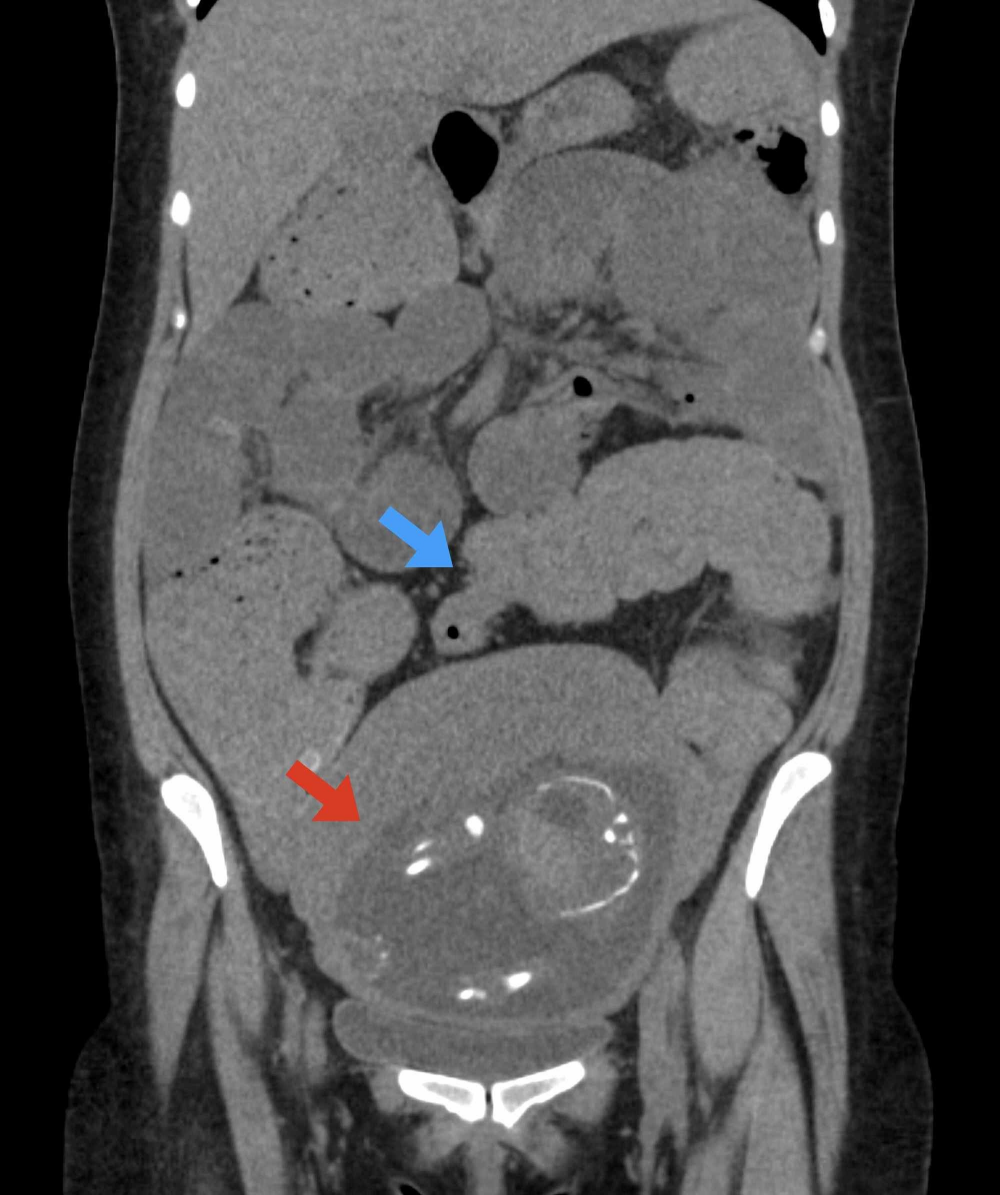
Coronal reconstruction from CT scan of the abdomen and pelvis demonstrating colonic distention with abrupt transition to complete collapse in the region of the sigmoid colon (blue arrow) and gravid uterus (red arrow)
